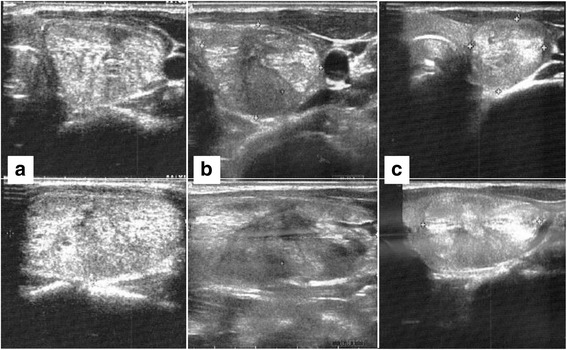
Ultrasound images showing the thyroid nodule (a) at the baseline, (b) 24 months after radiofrequency ablation, and (c) 12 months after laser ablation
